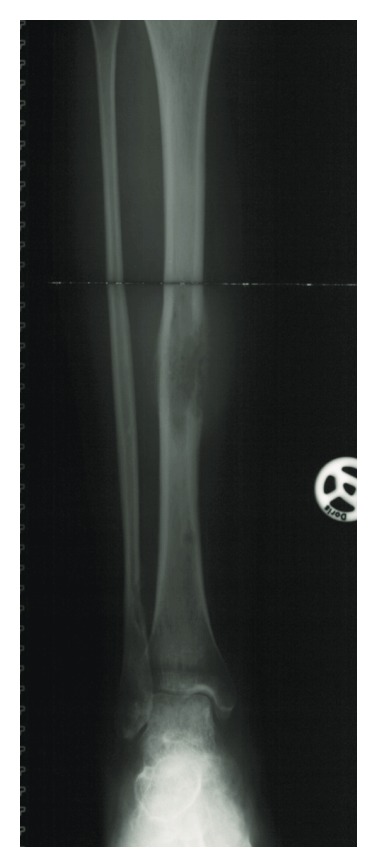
Preoperative AP radiograph of the right lower leg of a 23-year-old female patient with a multifocal adamantinoma of the tibia and the fibula.
